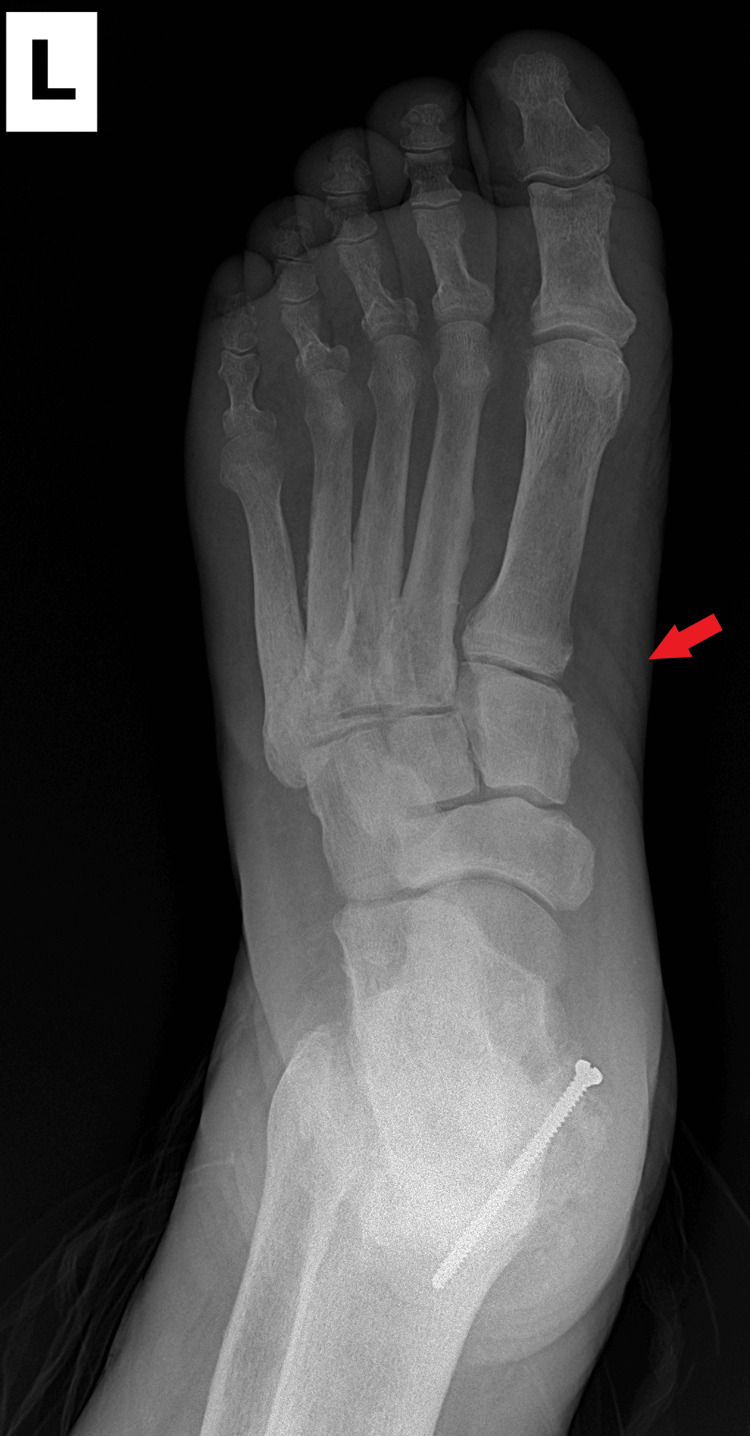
Anteroposterior radiograph of the left foot demonstrating soft tissue edema in the region corresponding to the area of green discoloration noted on physical examination (red arrow). An orthopedic screw is visualized in the medial malleolus.
